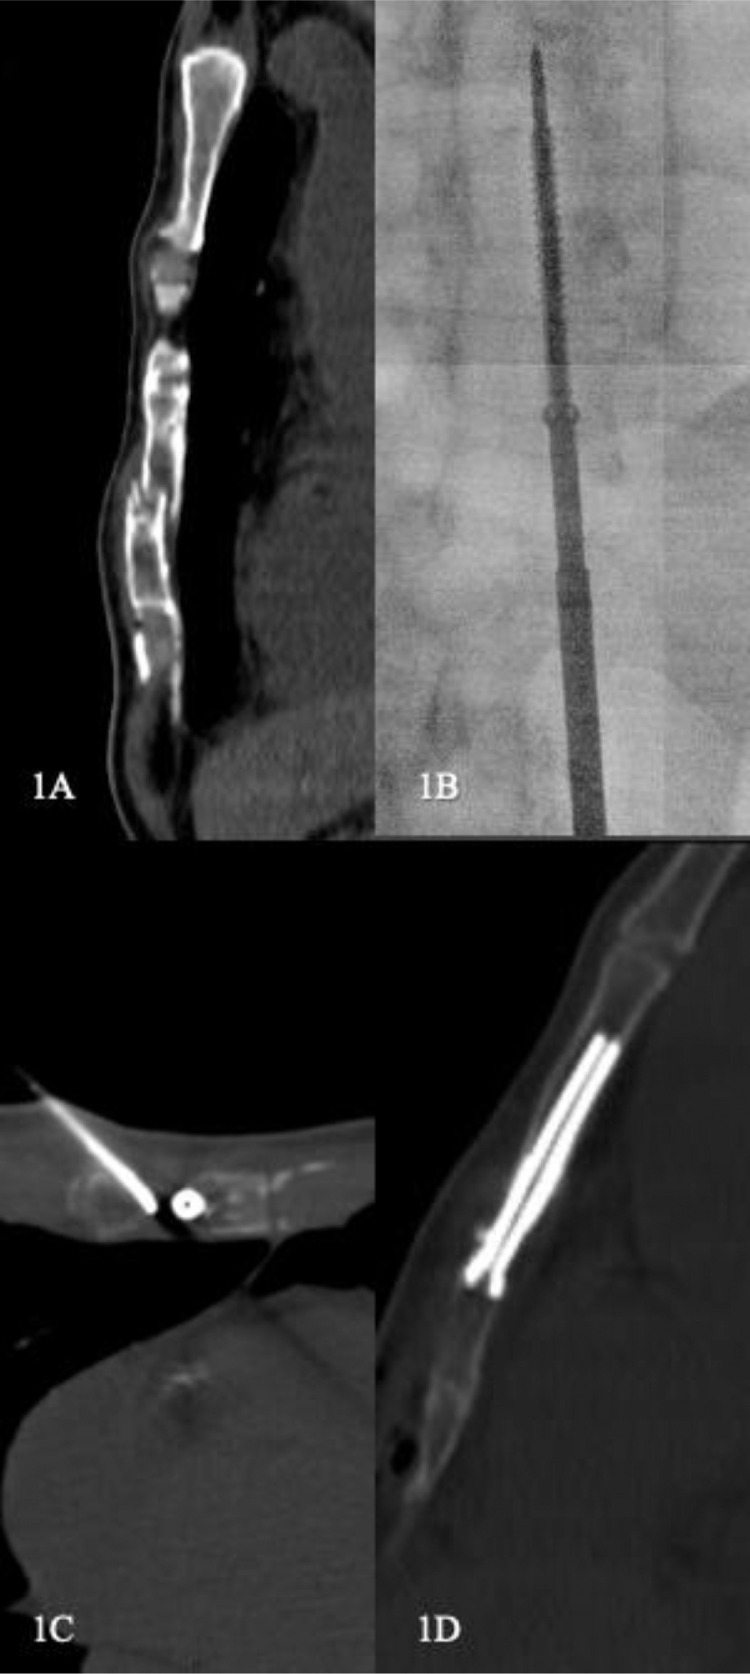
Sixty-six-year-old man with lung carcinoma, sternal metastasis, and a pathologic fracture. (A) Preoperative CT-scan images in sagittal section showing the pathologic fracture with 4 mm cortical displacement. (B) Frontal fluoroscopy showing the screw advance. (C) CT-scan in axial section showing the position of TCD trocar before cement injection (1C). (D) CT-scan sagittal section (1D) showing the result after the procedure, with no leaking of cement, satisfactory fracture stabilization, and no cortical effraction of the screws.
